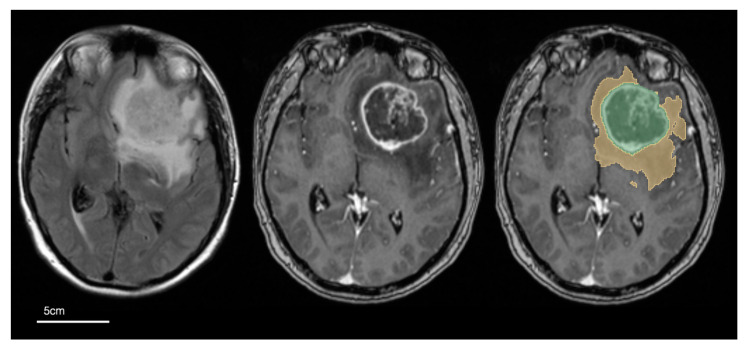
Definition of tumour volumes used in the study. Selected MRI axial images: left—fluid-attenuated inversion recovery (FLAIR); middle—gadolinium-enhanced T1-weighted (T1Gd); right—T1Gd image with overlay of core tumour segmentation (in green) and peritumoural oedema (yellow). Core volume (CV) is defined as the enhancing and necrotic component of the tumour (green) and whole volume (WV) is defined as the combination of core and peritumour oedema segmentation (green + yellow).
